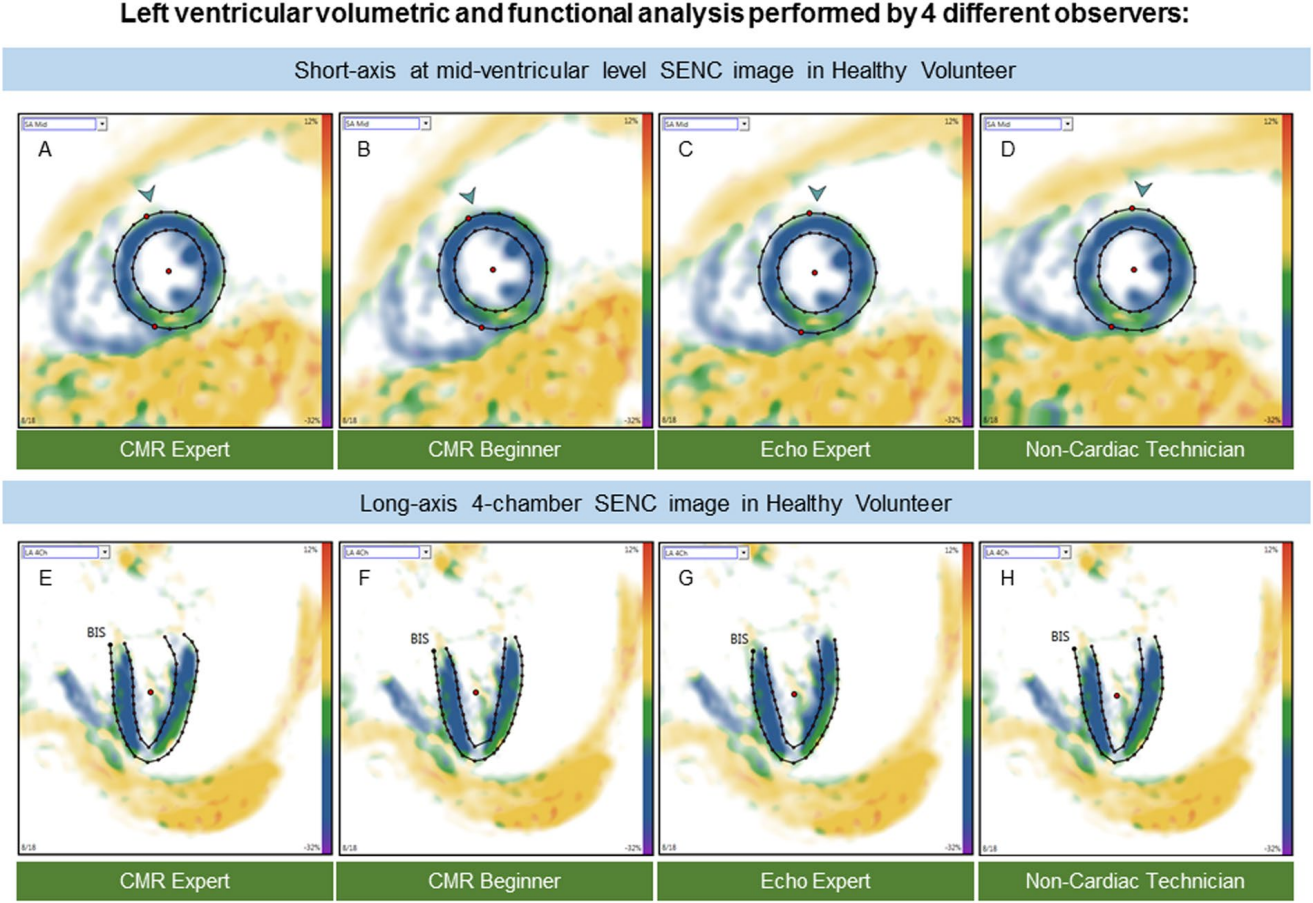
Example of fast-SENC images acquired in a healthy volunteer and uploaded into a dedicated MyoStrain software. CMR images were derived in three long-axis and three short-axis views. Endocardial and epicardial borders were traced at end-diastolic and end-systolic cardiac phases by four observers: CMR expert (A,E) CMR beginner (B,F) echocardiography expert (C,G) and non-cardiac technician (D,H). CMR = cardiac magnetic resonance; SENC = strain-encoded imaging.
